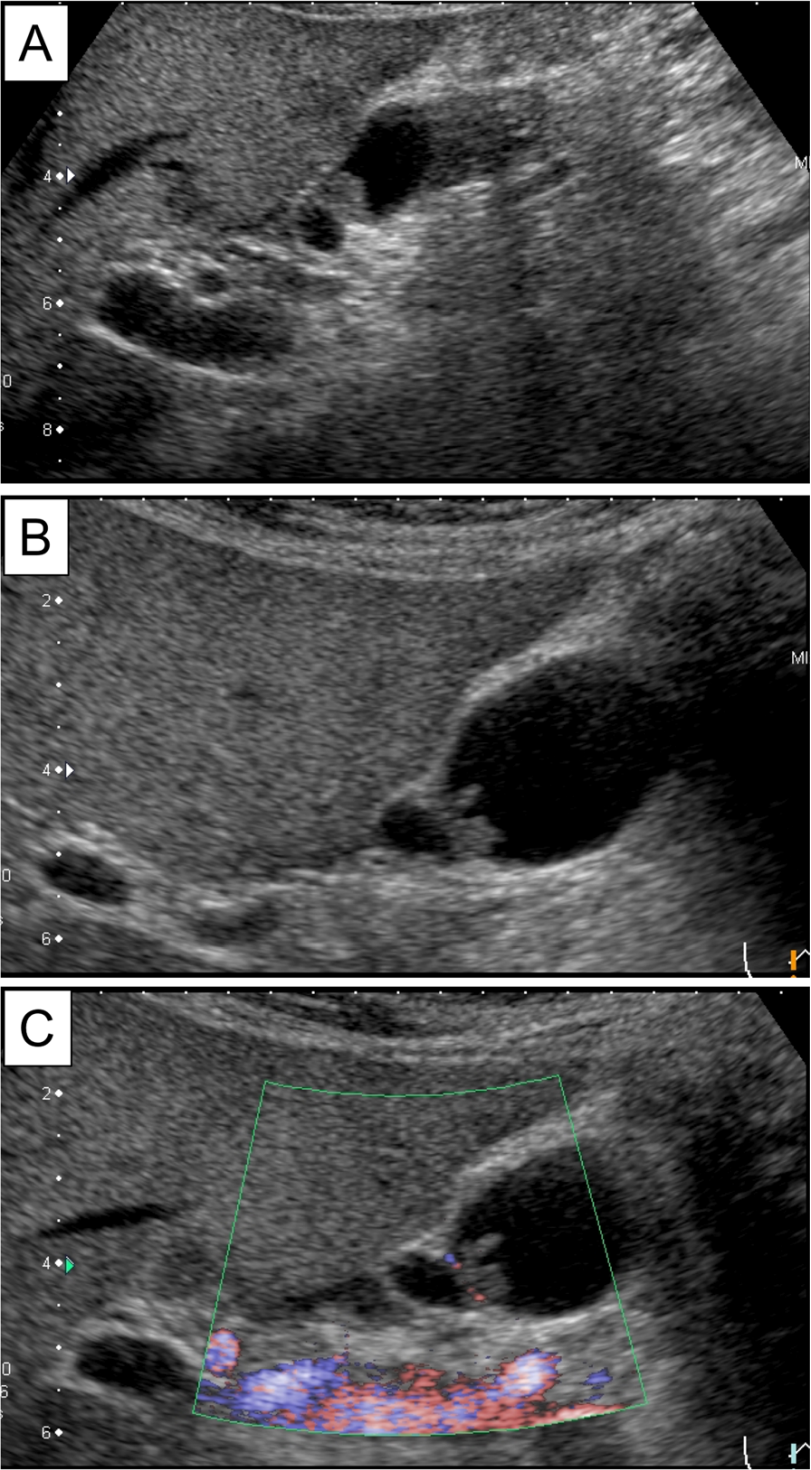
Representative images of the abdominal ultrasound. a A 10-mm polyp in the neck of the gallbladder was seen 6 months ago. b A 12-mm polyp in the neck of the gallbladder with perfusion and focal thickening of the gallbladder wall in the fundus. c Color Doppler imaging of the pedunculated polyp in the neck of the gallbladder
